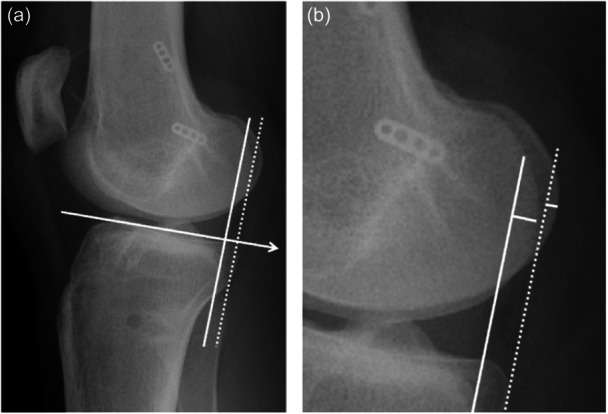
Radiographic measurements (anterior translation of tibia). (a) Anterior translation of the tibia measurement. A line was drawn along the subchondral plate of the tibial plateau (arrow). At the posterior margin of the medial and lateral tibial plateau, lines were drawn (solid line and dotted line) perpendicular to the first line (arrow). (b) The shortest distance from these lines to the most posterior cortical line of the femoral condyle was measured. Mean values for the medial and lateral sides were calculated.
